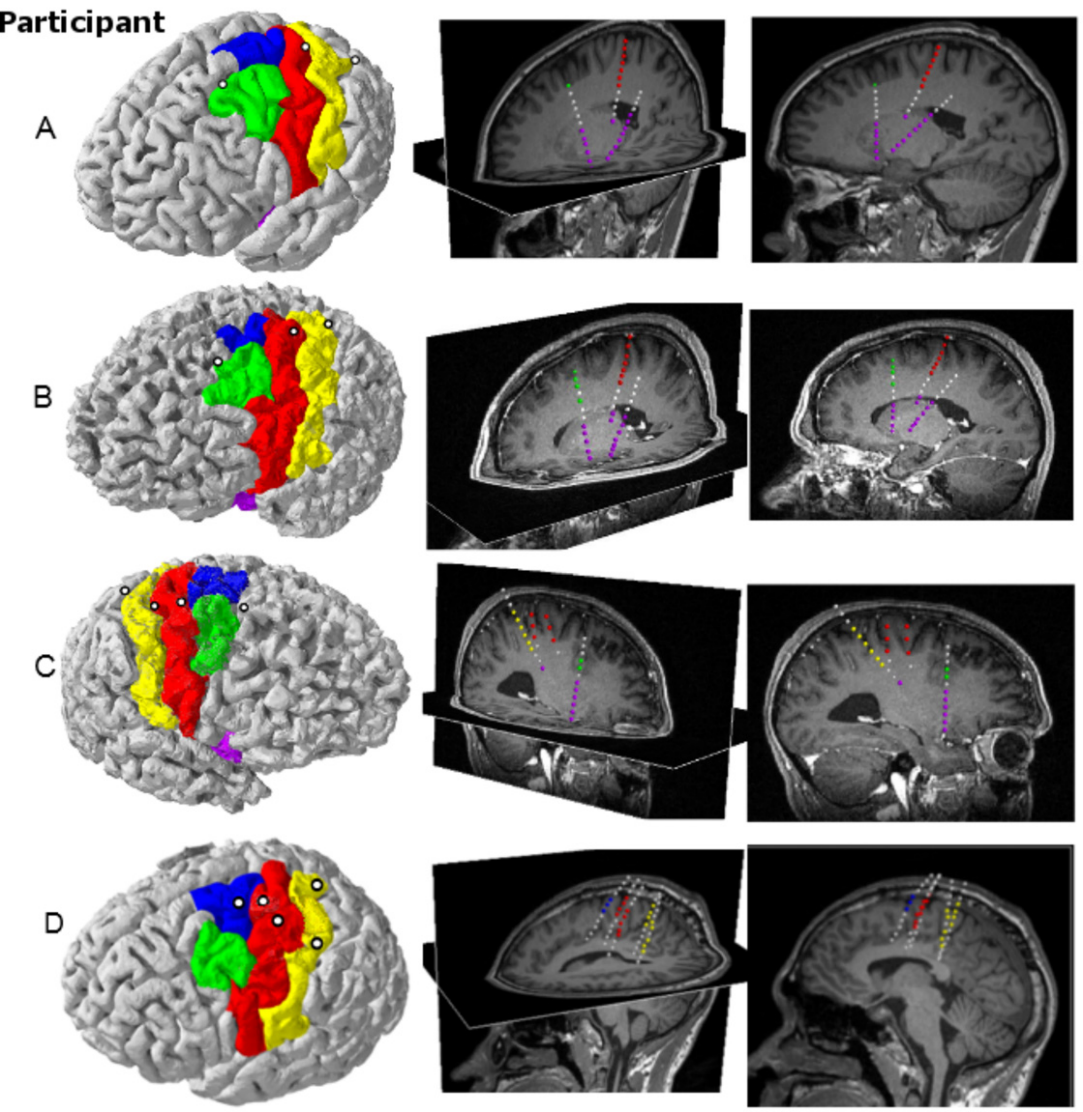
Locations of electrodes. Each row corresponds to an individual participant (A-D). *Left*: Participant specific cortical surface reconstructions (red: motor cortex, yellow: primary sensory cortex, green: premotor cortex, blue: supplementary motor cortex, purple: insular cortex) and electrode penetration sites (white circles). *Middle*: Oblique views of sagittal and axial MRI slices showing the electrode trajectories. Electrode contacts are color-coded according to the cortical region they pass by or through. The MRI slices shown are located as close to the electrodes as possible without obscuring any of the contacts. *Right*: Sagittal views showing the actual depths of the electrodes.
